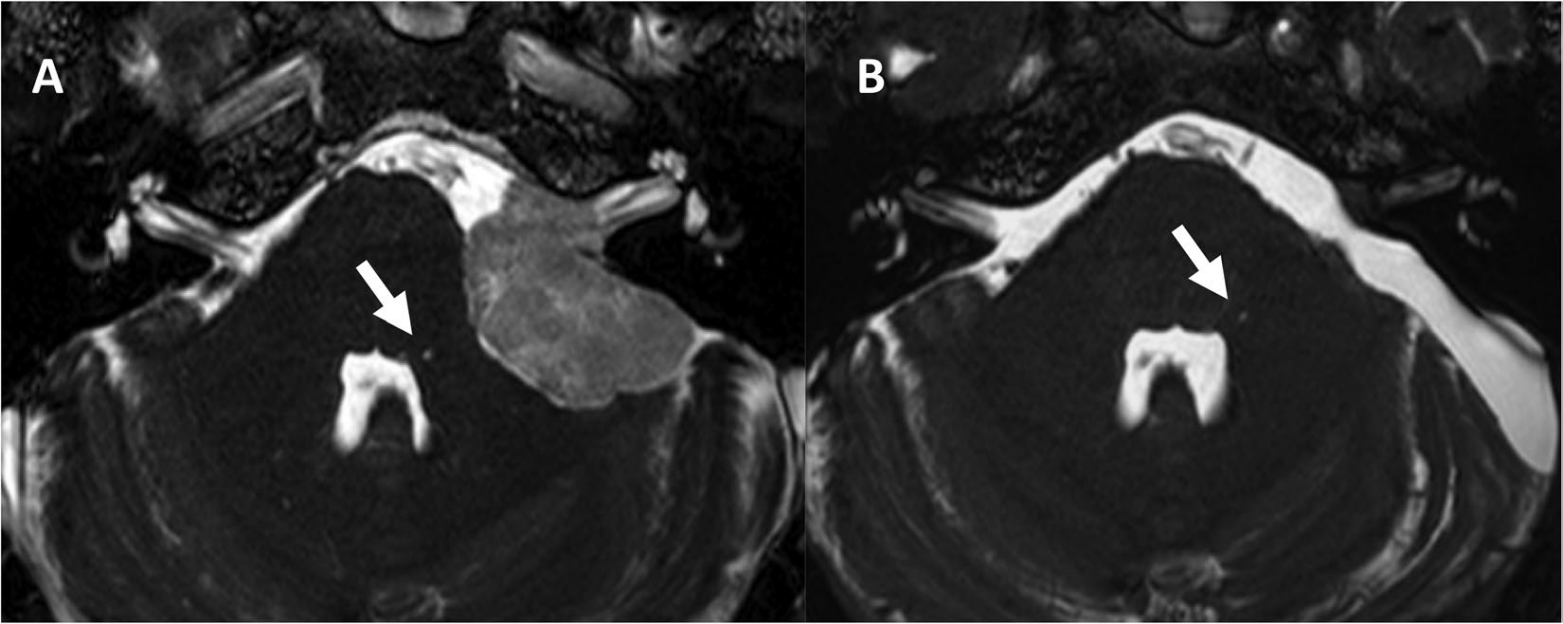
Representive preoperative Gd-enhanced b-FFE MR image of the FHI (arrow) in a patient with cerebellopontine angle meningioma (A). Postoperative (B) b-FFE image shows complete resection of tumor and no signal change of FHI (arrow).
